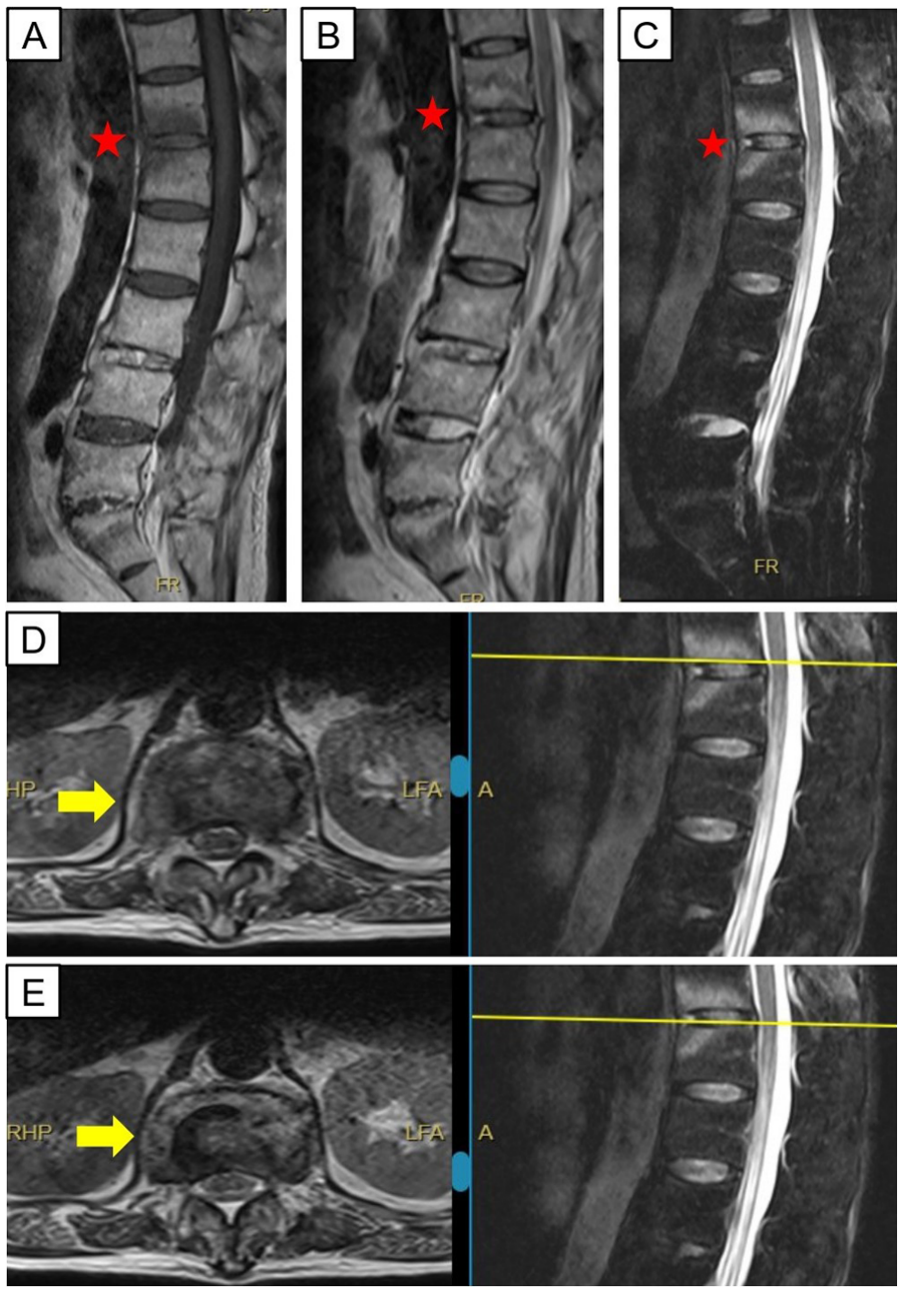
Magnetic resonance images of the patient before admission. The red pentagrams indicate the lesion mimicking ModicⅠchanges, which are hypointense on T1-weighted sequences (A), hyperintense on T2-weighted sequences (B) and STIR sequences (C) Yellow arrows show inhomogeneous signal in the disc space at T12/L1 in keeping with discitis (D,E).
